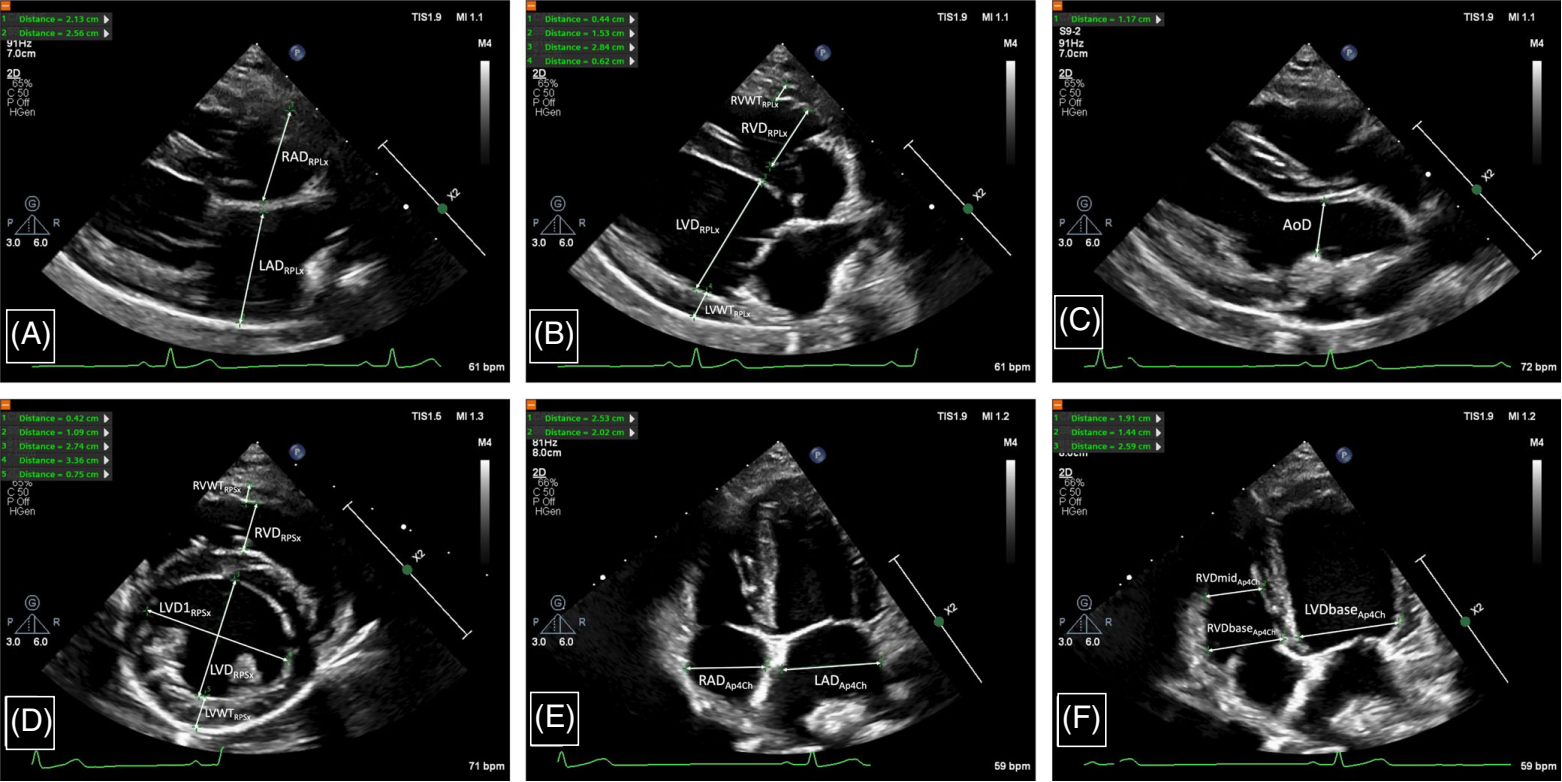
Example measurements of right atrial dimension (RAD) and left atrial dimension (LAD) from a right parasternal long‐axis (RPLx) 4‐chamber view at end‐systole (A), right ventricular wall thickness (RVWT), right ventricular dimension (RVD), left ventricular dimension (LVD), and left ventricular wall thickness (LVWT) at end‐diastole (B), aortic valve diameter (AoD) in early to midsystole measured between the hinge points of the maximally opened aortic valve cusps from a right parasternal long‐axis view optimized for the left ventricular outflow tract and ascending aorta (C), RVWT, RVD, cranio‐caudal LVD, latero‐lateral left ventricular dimension (LVD1), and LVWT from a right parasternal short‐axis view (RPSx) optimized for the high papillary muscles/chordal attachments at end‐diastole (D), RAD and LAD from a left apical 4‐chamber view (Ap4Ch) with the beam centered on or just to the left of the interventricular septum at end‐systole (E), and, in the basilar one third of the chamber, right ventricular dimension (RVDbase) and left ventricular dimension (LVDbase), and right ventricular dimension at the midchamber level (RVDmid) from a left Ap4Ch view at end‐diastole (F). See the text for further details on performing these measurements.
